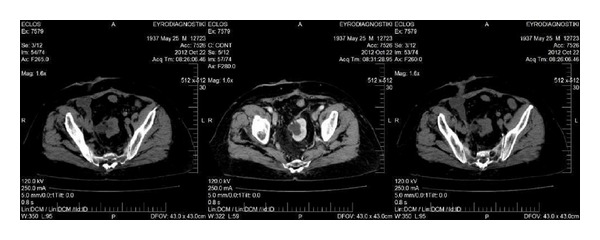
Postoperative CT of case  1.
